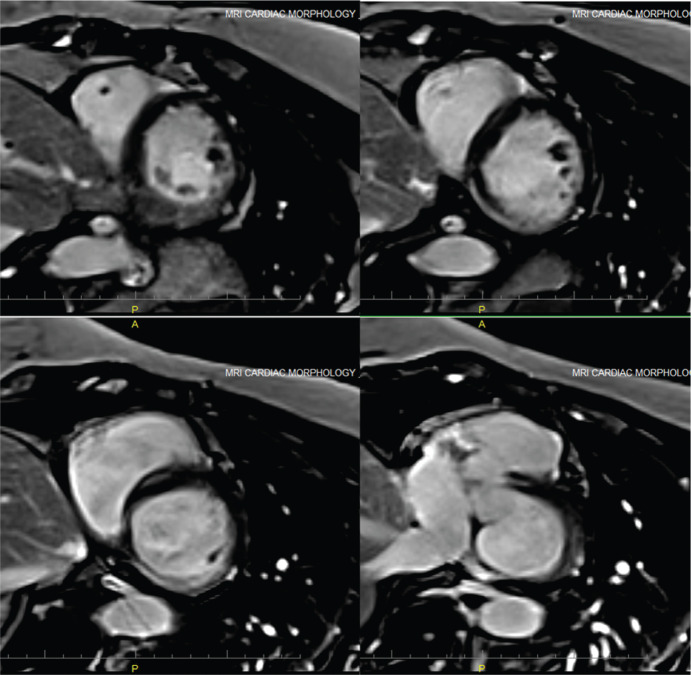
Cardiac MRI scan. Delayed enhancement shows midmyocardial/epicardial scar involving the basal-inferior and inferoseptal walls.
